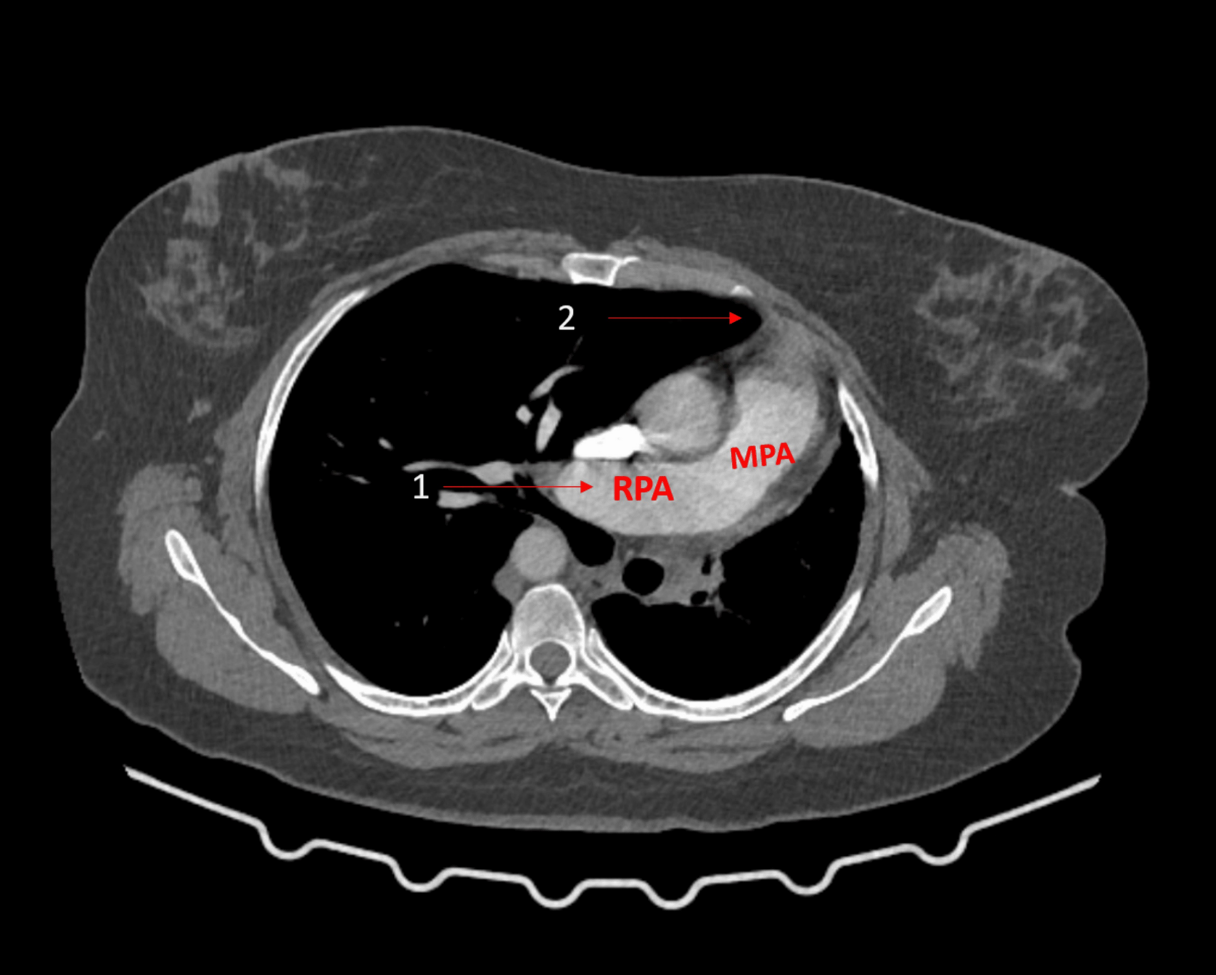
CT pulmonary angiogram 1 shows the right pulmonary artery (RPA) and main pulmonary artery (MPA). 2 shows the herniation of the right lung.
